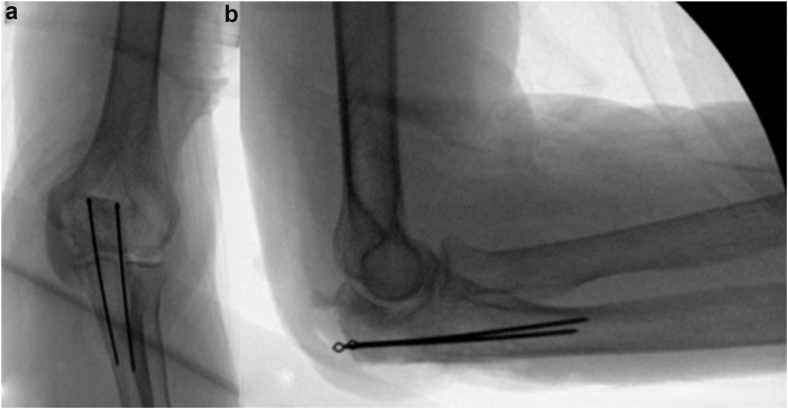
Post-operative radiographs of the final construct demonstrating appropriate reduction. (a) AP and (b) lateral radiographs.
